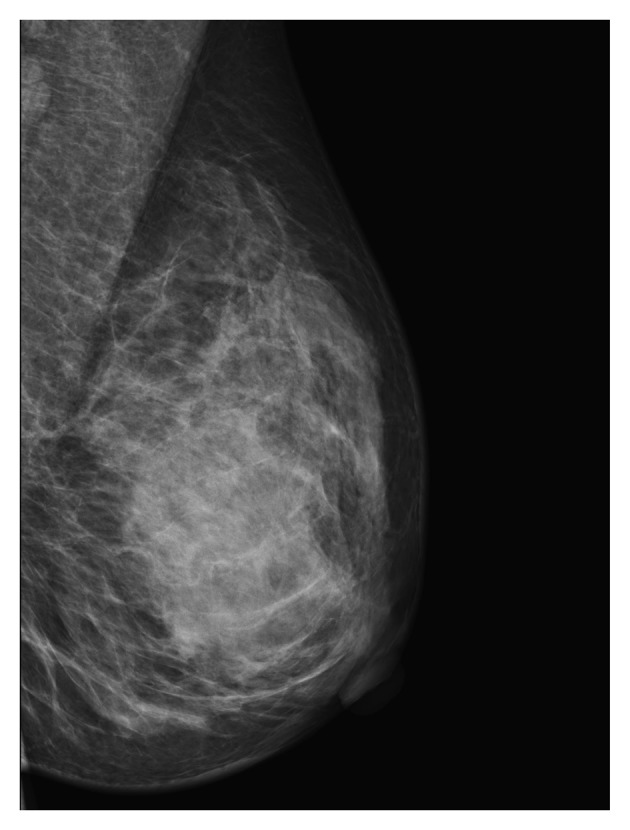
Digital radiography examination revealed a 5.0×4.0×4.0 cm mass with an increased density shadow in the left breast.
